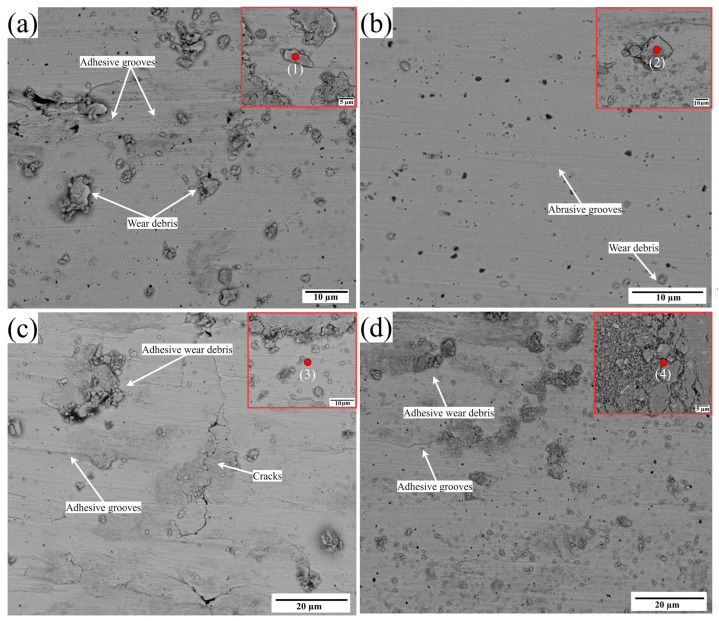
SEM morphology and EDS analysis of wear surfaces and wear debris (a) without ultrasonic with point EDS of subset at point 1 and with ultrasonic vibration amplitudes of (b) 5 µm with point EDS of subset at point 2, (c) 10 µm with point EDS of subset at point 3, and (d) 15 µm with point EDS of subset at point 4.
